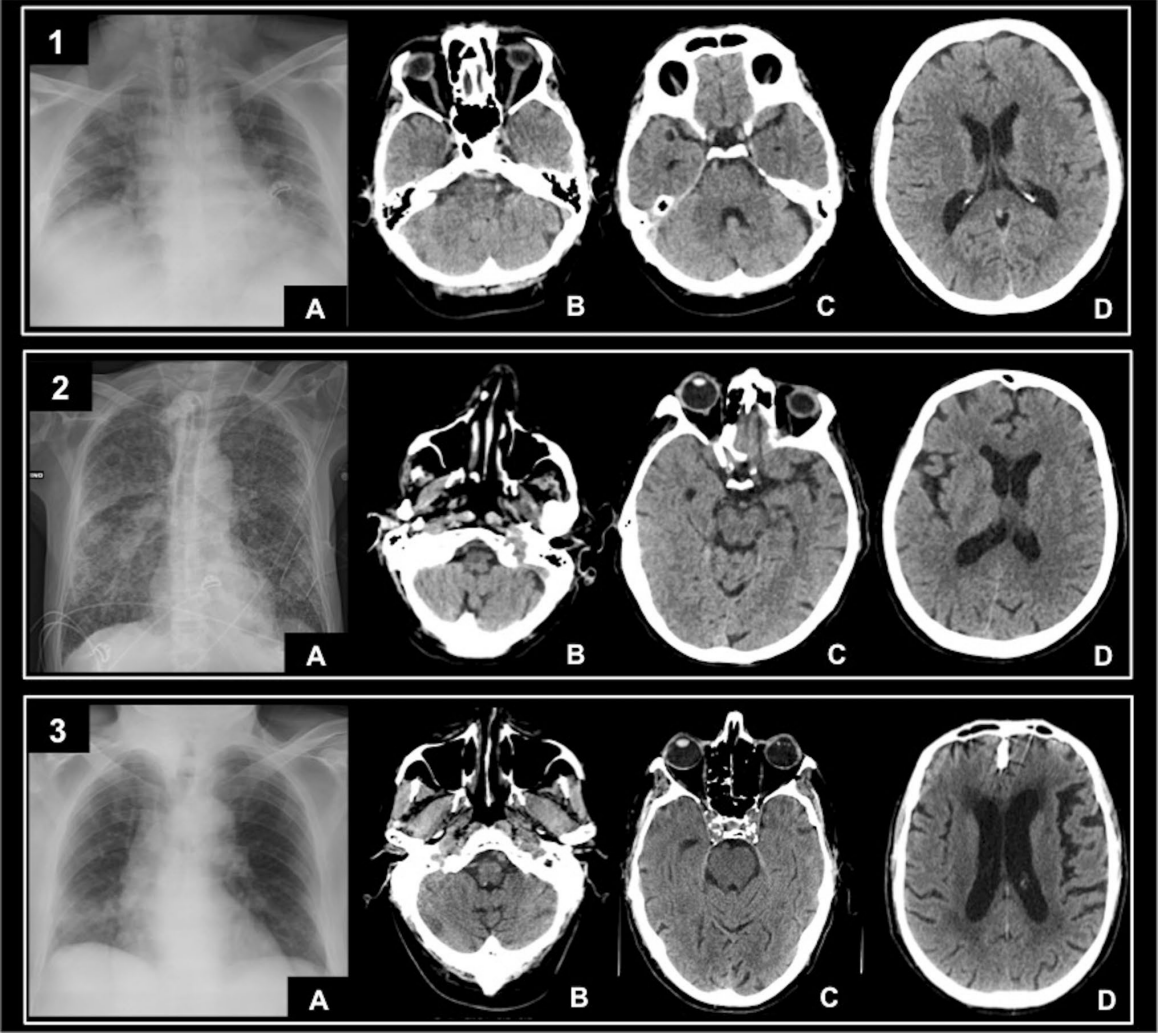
Panel 1: Man, 57 years old. The CXR showed advanced lung disease with diffuse consolidations and interstitial involvement: RALE 20 [A]. The brain CT scan was performed for syncope and head trauma in patient with fever. Only the presence of elongation of the vertebral artery (index D, [B]) was found on CT scan by both observers. The CVD-CT score was 0. The patient didn’t need invasive ventilation and survived. Panel 2: Man, 69 years old. The CXR showed severe lung disease with diffuse consolidations and interstitial involvement: RALE 30 [A]. The brain CT scan was performed to exclude possible brain lesions in a sedated patient with prolonged mechanical ventilation. Both observers agreed on the presence of elongation of the vertebral artery (index D, [B]) and vessel calcifications of both carotid siphons (index C, [C]). The CVD-CT score was 3. The patient survived. Panel 3: Man, 76 years old. The CXR showed moderate lung disease with diffuse consolidations and interstitial involvement: RALE 14 (A). The brain CT scan was performed for disequilibrium in patient with fever. Both observers agreed on the presence of elongation of the vertebral artery (index D, [B]) and vessel calcifications of both carotid siphons (index C, [C]). Moreover, they agreed on the presence of severe leukoaraiosis [D]. The CVD-CT score was 5. The patient needed invasive ventilation but died during hospitalization
